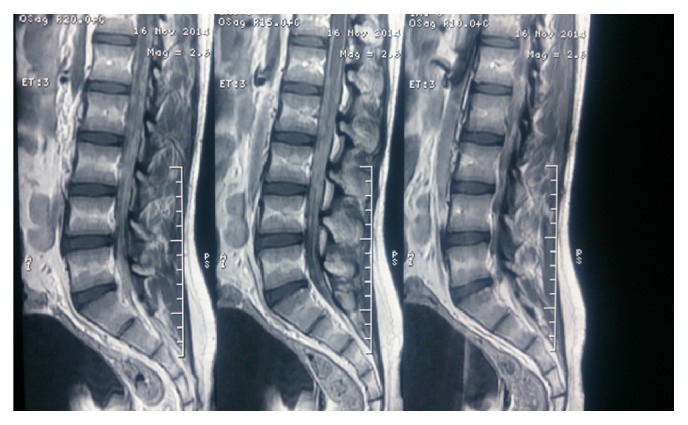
Spine MRI showing drop metastases in the lumbosacral spine.
